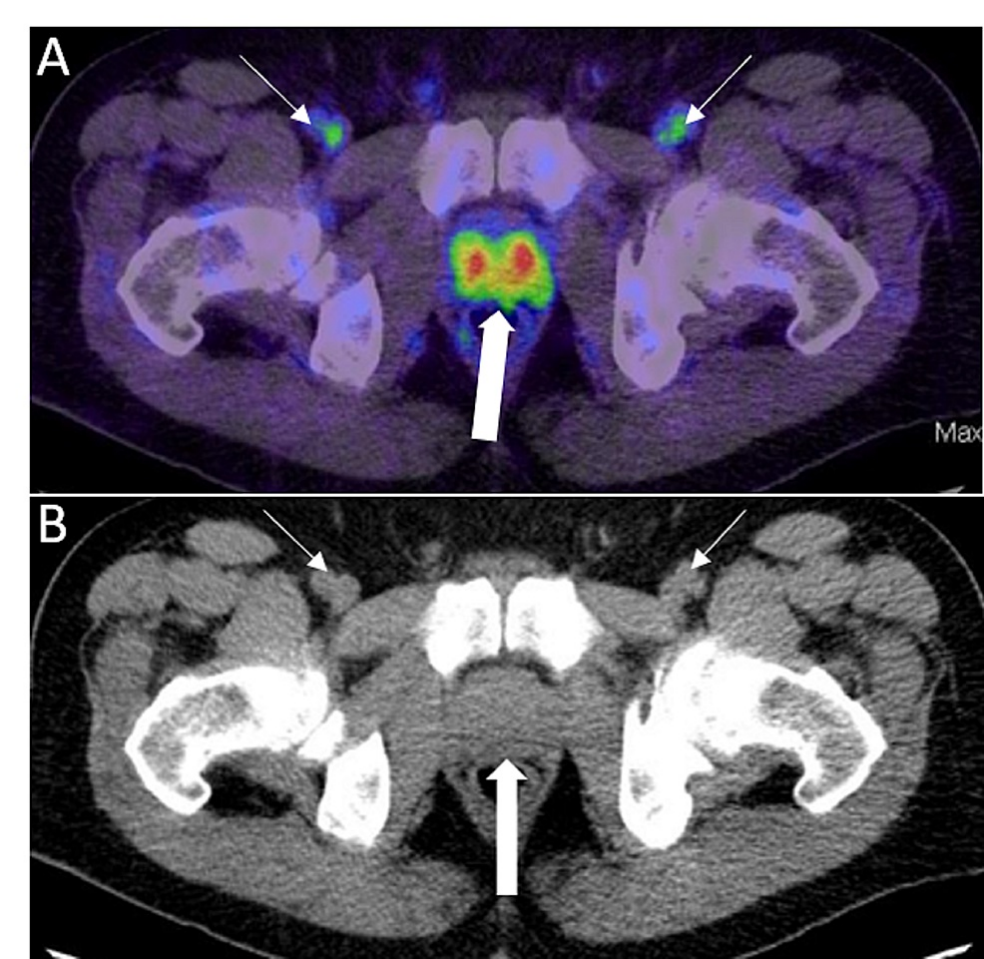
PSMA-RADS-2 A: Axial 68Ga PSMA PET/CT image through the pelvis shows subcentimeter bilateral inguinal lymph nodes with mild uptake (white thin arrows). There was no other pelvic lymphadenopathy in this patient. Note the intense radiotracer uptake in the primary prostate cancer (white thick arrow). B: Axial CT image through the pelvis in the same patient shows correlate subcentimeter bilateral inguinal lymph nodes (white thin arrows). Note the enlarged prostate (white thick arrow).
